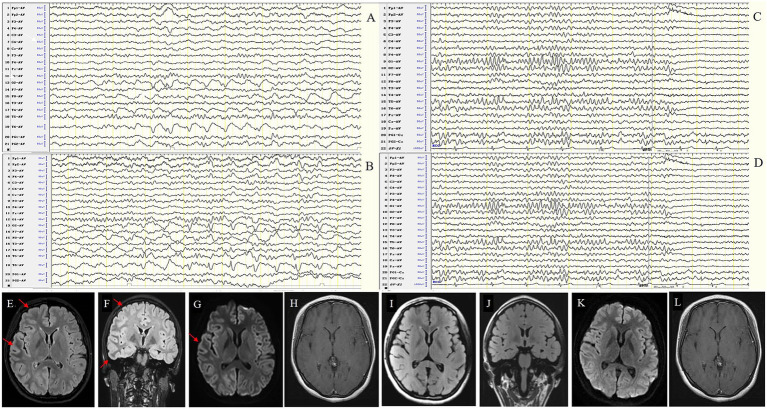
Case 54, female, 16 years old, who was diagnosed with MOG antibody cortical encephalitis (FLAMES). (A,B) Twenty-one days after onset and before immunotherapy, EEG showed diffuse slow waves in the right hemisphere in background activity (A) and interictal epileptic discharges in the right occipital and posterior temporal regions (B). MRI shows swelling of the left frontotemporal cortex (E–H). (C,D) Ninety days after immunotherapy, EEG showed significant improvement in background activity without interictal epileptic discharges. MRI showed that the swelling of the left frontotemporal cortex was improved (I–L).
